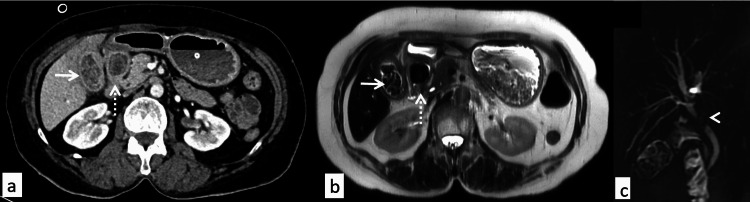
Cross-sectional images (A) Axial contrast-enhanced CT images showing multiple gallstones in the thickened gallbladder (arrow) with thickening of adjacent duodenum containing hyperdense contents (dashed arrow). (B) Axial T2-weighted MRI confirming the presence of gallstones (arrow) and large hypointense stone within the duodenal lumen (dashed arrow). (C) Magnetic resonance cholangiopancreatography image showing mild biliary dilatation with signal loss (arrowhead) due to extrinsic compression in proximal common bile duct resulting in Mirizzi syndrome.
